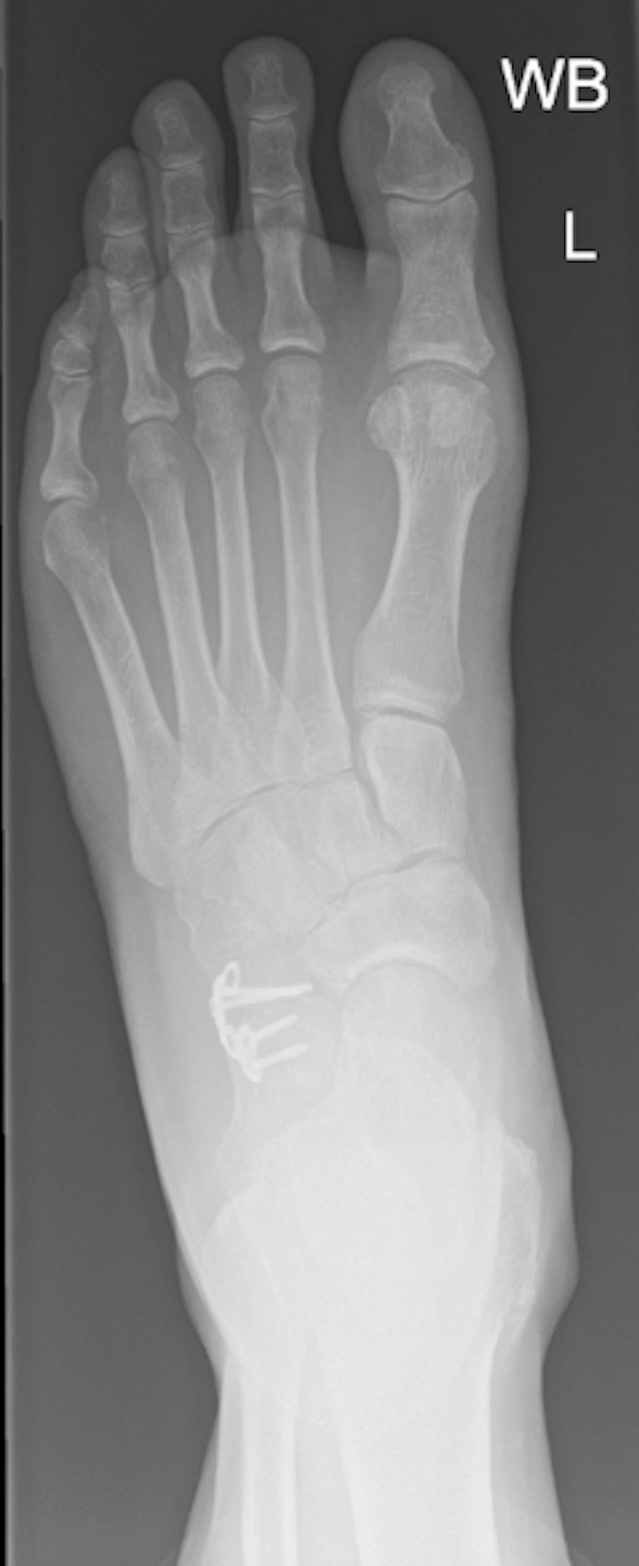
Anteroposterior radiograph of the left foot at four months post-operative
